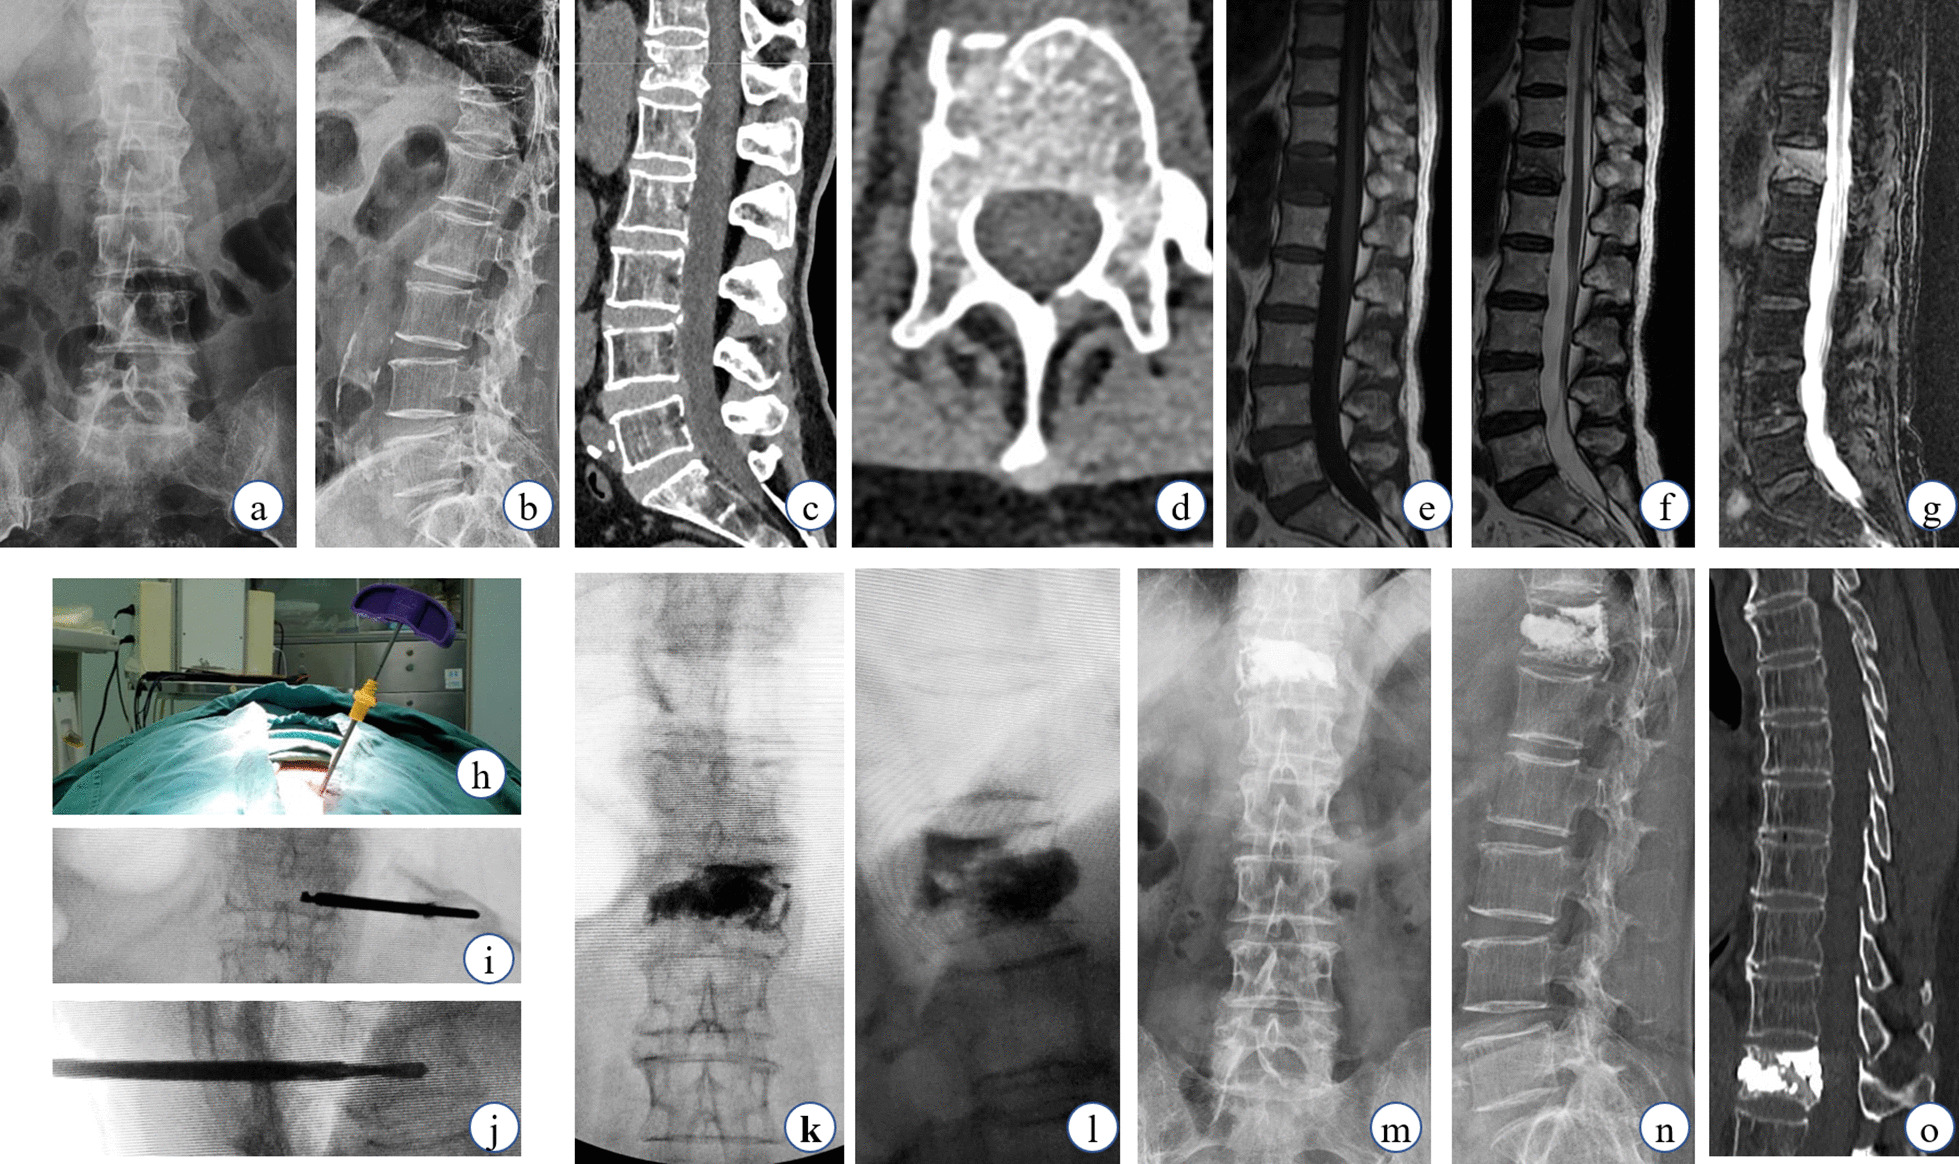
The clinical data of a 72-year-old female OVCF patient with UTTP PKP surgery. The fresh fracture was located at T12 by X-Ray (a, b), CT scan (c, d) and MRI (eg). And it could be also observed from CT scan that the anterior wall was broken in this vertebra. Forty-degree extraversion angle was used (h) and the tip of trocar reached the midline area (i, j). T12 vertebra was almost filled up with cement on the fluoroscopy and a little cement leaked from the anterior wall of vertebral body (k, l). Symptom relief and correction of spinal deformity were taken effect immediately after surgery. At the follow-up one month later, symptom was further relieve and no re-collapse was observed (mo)
